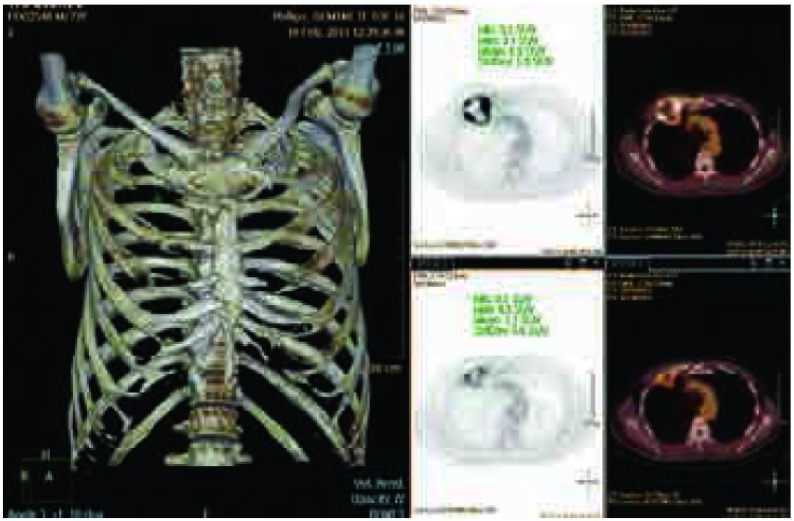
病例10，男性，74岁，右肺上叶癌，侵犯右第2前肋及胸小肌，实施新辅助化疗2周期后手术。术前CT三位骨重建明确显示右第2前肋受累，与化疗前PET比较，局部病灶最大SUV值由10.8降至7.7。 Case 10, 74-year old male, with lung cancer of right upper lobe invading 2^nd^ rib and pectoralis minor muscle. Treated with 2 cycles of neo-adjuvant chemotherapy followed by surgery. Three-dimensional CT synthetic image shows tumor invading 2^nd^ rib, compare with the first PET/CT prior to chemotherapy (top right). SUV decreased from 10.8 to 7.7. SUV: standard uptake value.
